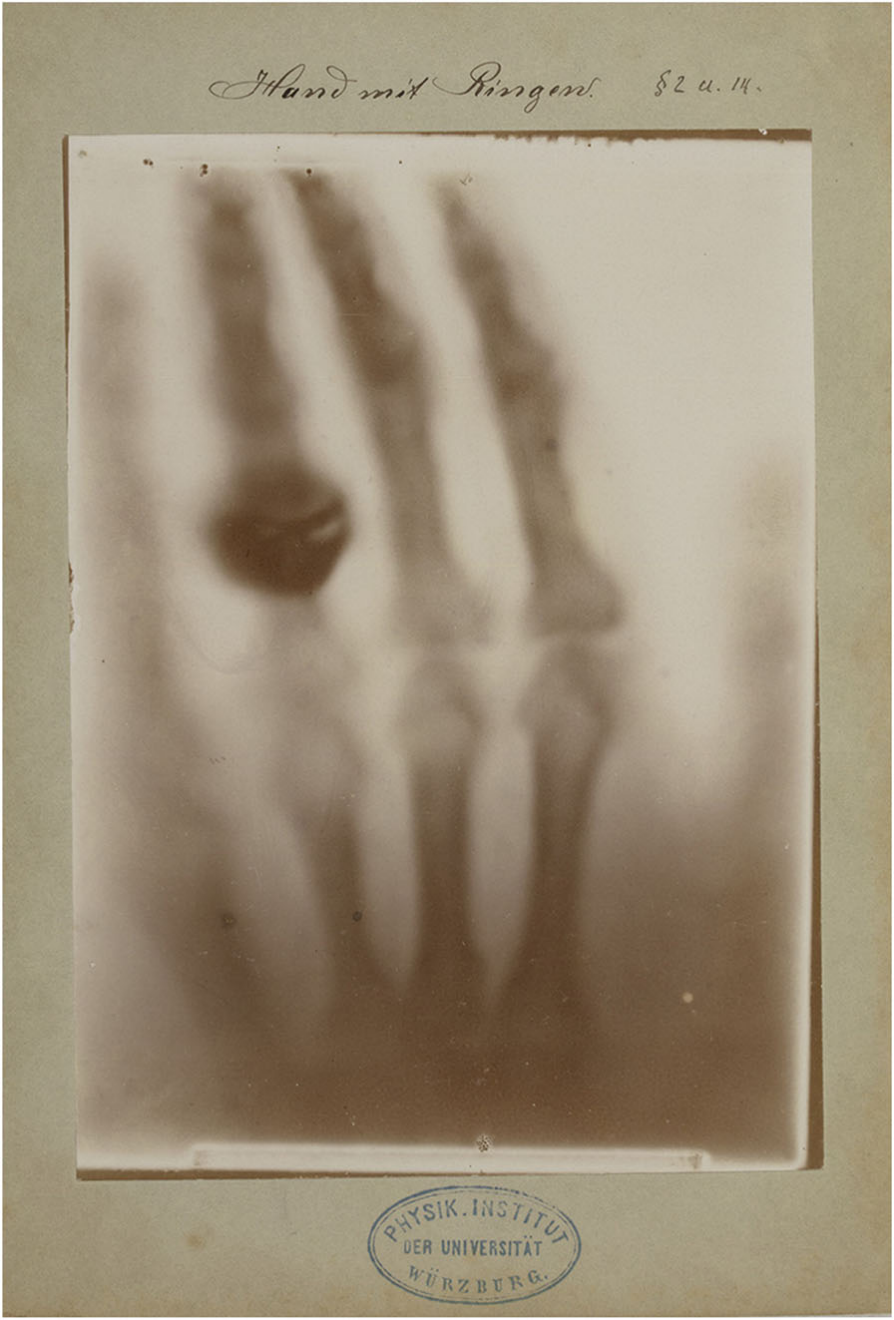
The most impressive radiographic image is the one taken on December 22nd, 1895 of Bertha’s hand as it is the only image which shows the importance of X-rays for medicine as it is the first anatomical radiograph. It is therefore seen as the beginning of radiography. For *Time Magazine*, it was the reason to nominate it as one of the 100 most influential photographs ever. The other eight radiographs are of a technical nature. Manually written legend: ‘Hand mit Ringen’ (Hand with rings). Paragraph referral: § 2 and § 14
